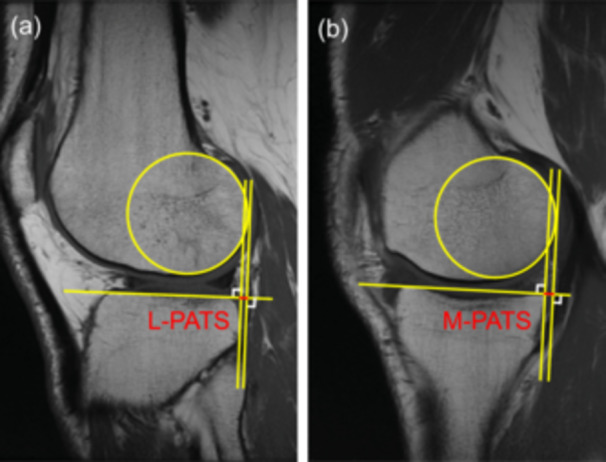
Magnetic resonance images demonstrating the measurement of passive anterior tibial subluxation (PATS) (red segments). (a) Lateral PATS (L‐PATS) was measured on the midsagittal slices of the lateral compartment showing the medial aspect of the fibula head. (b) Medial PATS (M‐PATS) was measured on the midsagittal slices of the medial compartment showing the femoral insertion of the medial gastrocnemius tendon.
